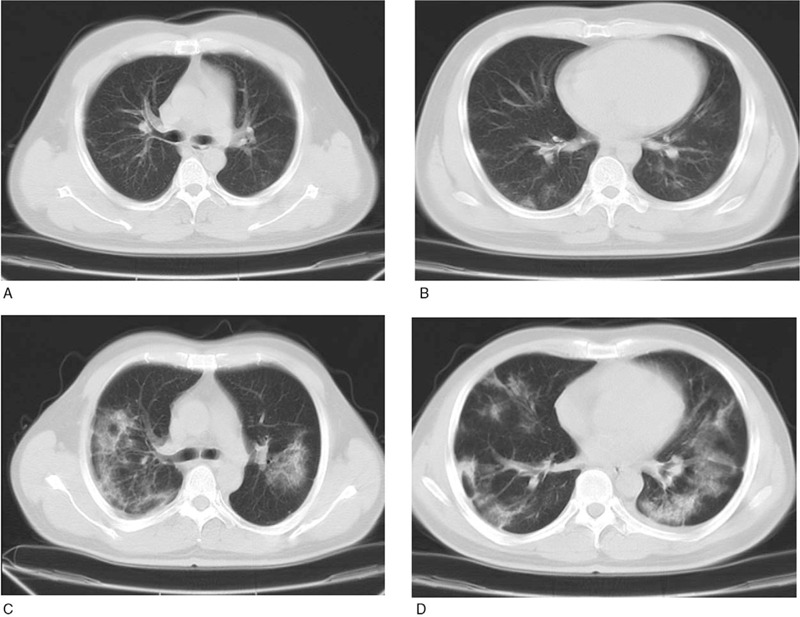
Imaging characteristics of chest computed tomography from common type of coronavirus disease 2019 (COVID-19) and severe type patients. A and B, 35-year-old male with common type COVID-19 patient exhibited multiple ground-glass opacities in both lungs. C and D, 49-year-old male with severe type COVID-19 exhibited diffuse ground-glass opacities and consolidation distributed in multiple lobes and segments.
